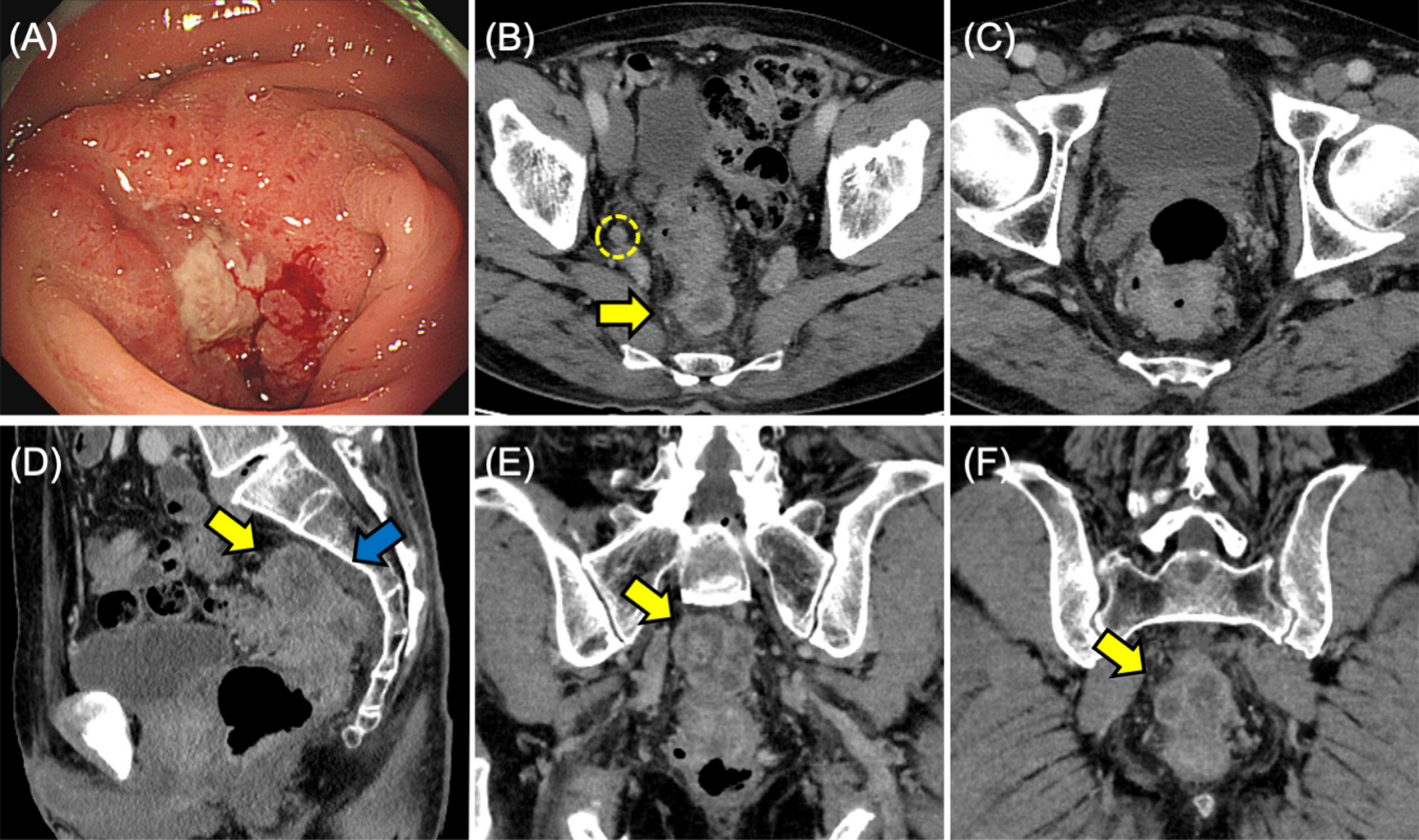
Initial diagnostic images of the rectal tumor before treatment. (A) Colonoscopy showing a circumferential tumor of the lower rectum. (B–F) Computed tomography demonstrating a rectal tumor with extramural infiltration (yellow arrows) accompanied by fluid collection (blue arrow) and lateral lymph node adenopathy (yellow dotted circle).
